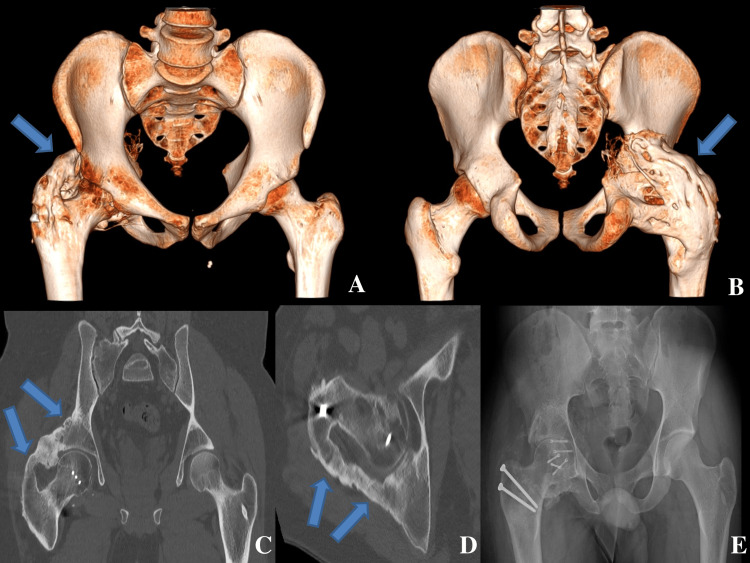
Pre- and postoperative imaging of the first patient A and B: preoperative pelvis 3D-scan image; C: coronal view of the preoperative pelvis multiplanar reconstruction (MPR); D: axial view of the preoperative pelvis MPR; E: postoperative pelvis X-ray
